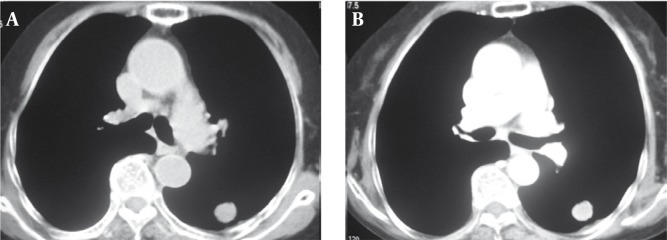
Computed Tomography of a 66-Year-Old Man Presenting With Chest Pain and Cough. A, Plain CT scan shows a round mass in the left lung. B, Enhanced CT scan shows slight homogeneous enhancement in the mass which was verified to be lung cancer.
